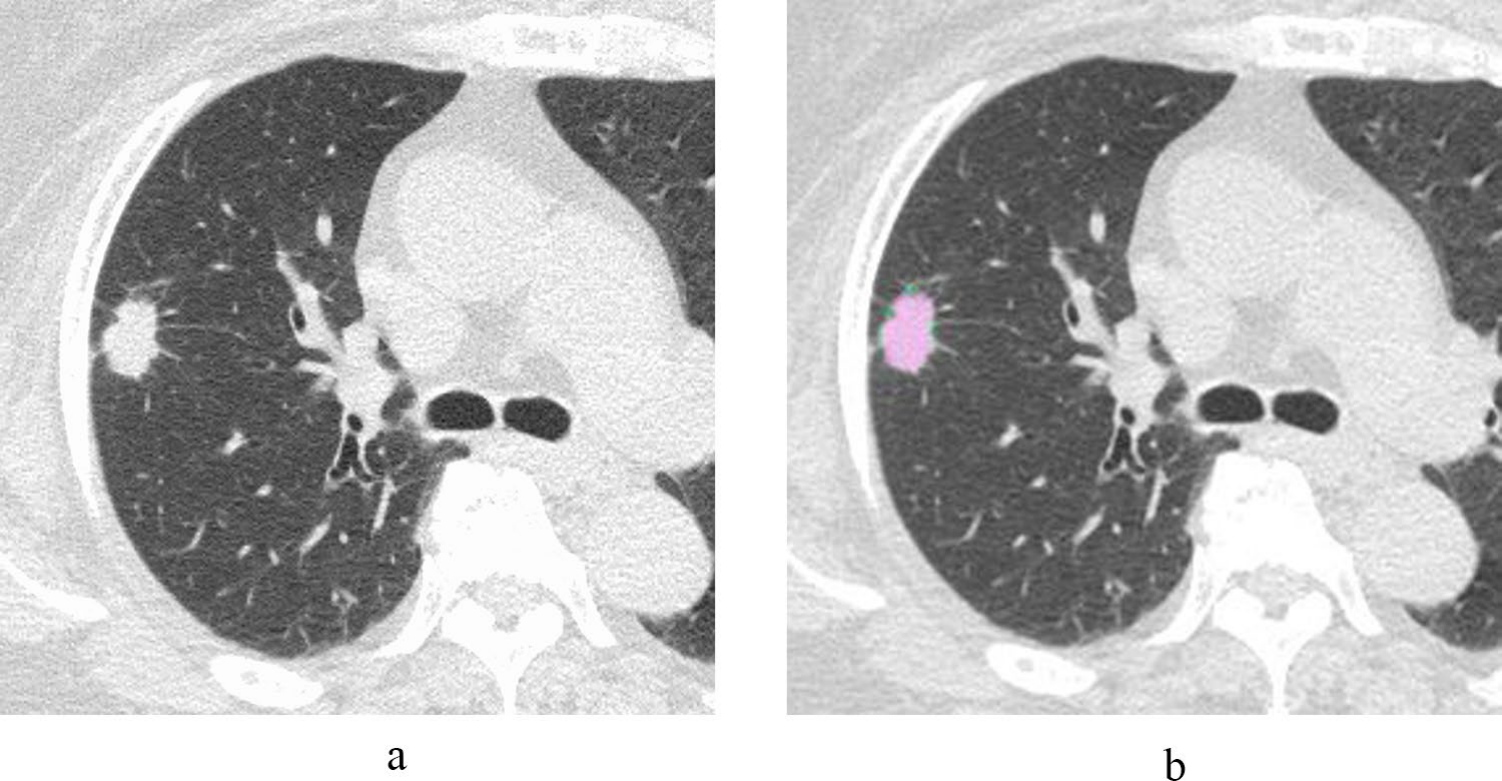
A 68-year-old woman with invasive adenocarcinoma (TPS ≥ 50%). a HRCT shows a solid nodule. The diameters of both the tumor and solid component are 17.7 mm. The rate of the solid component diameter is 100%, b The volume of the tumor and solid component are 2206.8 mm^3^ (pink and green area) and 2195.1 mm^3^ (pink area), respectively. The rate of the solid component volume is 99.5%. *TPS* tumor proportion score, *HRCT* high-resolution CT
